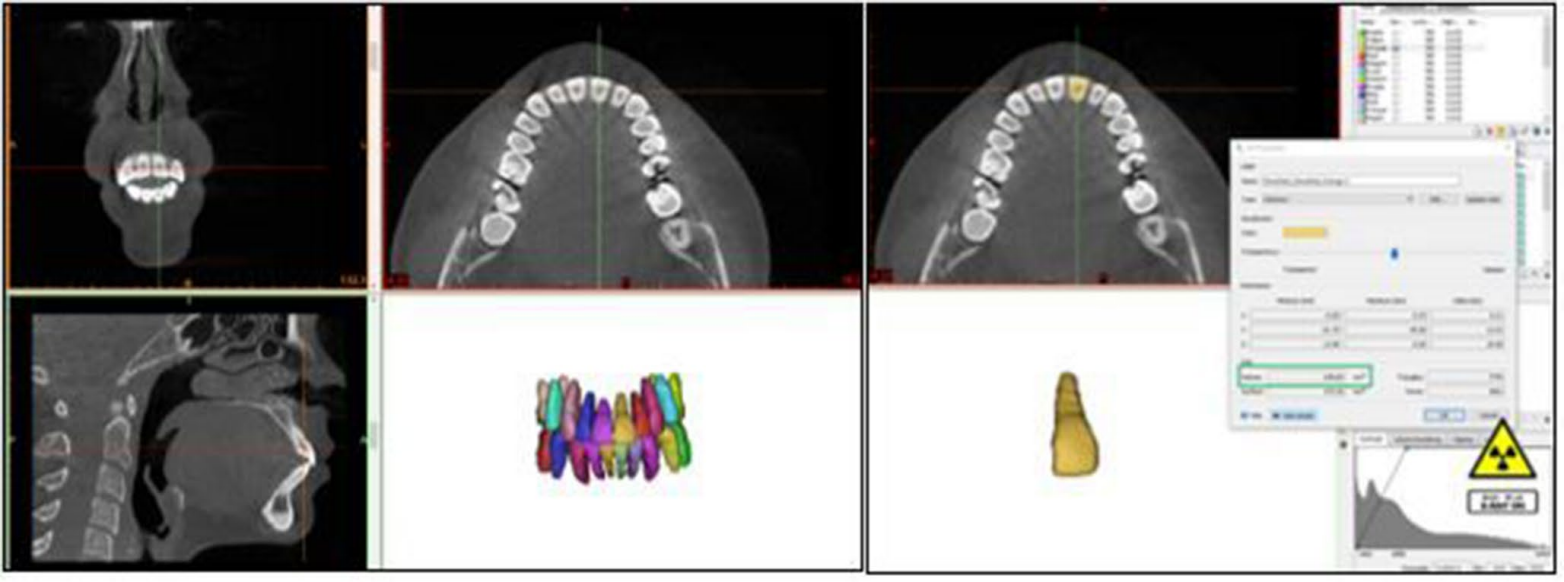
Three-dimensional segmentation and volumetric analysis of tooth roots using CBCT images
